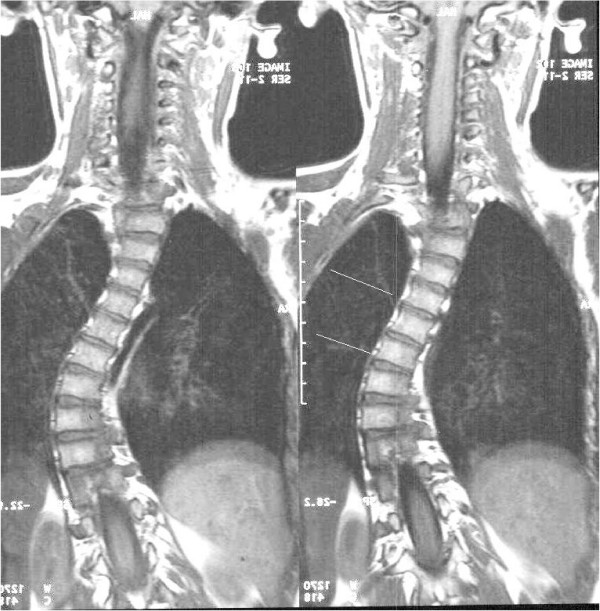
MRI T1-weighted Sequence, Coronal Image. Idiopathic Scoliosis Patient #3. Superior line indicates central zone deficiency and inferior line indicates convex zone growth plate deficiency.
